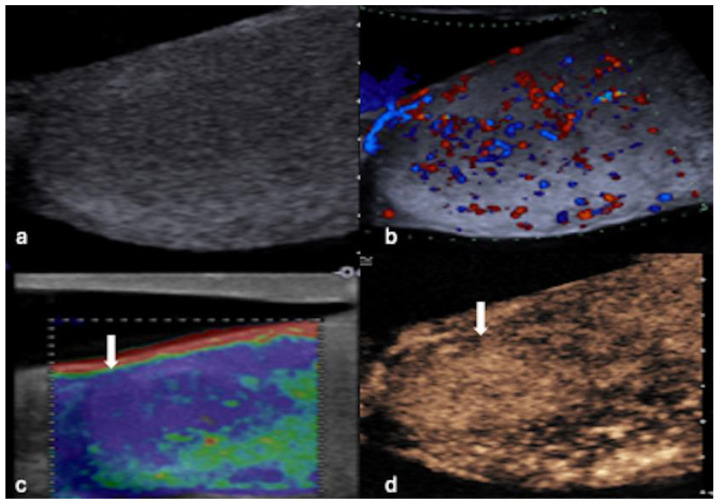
MPUS of a testicular lymphoma. (a) Greyscale ultrasound shows diffuse enlargement of the testis with ill-defined, extensive decreased echogenicity in the majority of the testis. (b) CDUS indicates that hypervascularity is present within the testis. (c) SE demonstrates a hard lesion (white arrow), which is not clearly depicted on greyscale US. (d) CEUS demonstrates hyper-enhancement of the lesion (white arrow).
